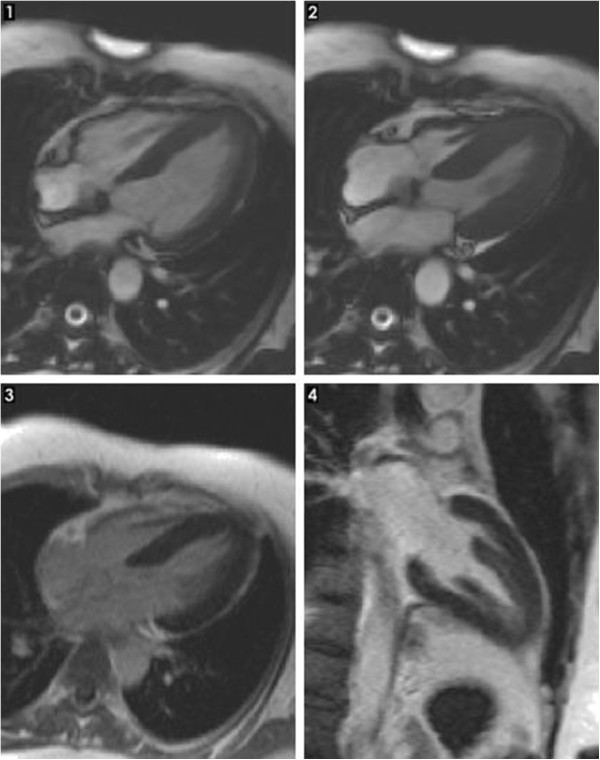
Cardiac MRI: 1,2 cine steady state free precession (SSFP) in four chambers view with apical ballooning; 3,4 triple inversion-recovery post injection of contrast agent with no late enhancement in the left myocardium.
